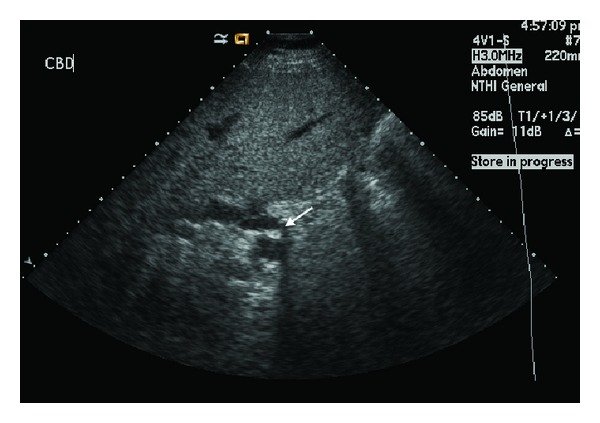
Transabdominal ultrasound of common bile duct (CBD) with CBD stone (arrow) and associated posterior acoustic shadowing.
